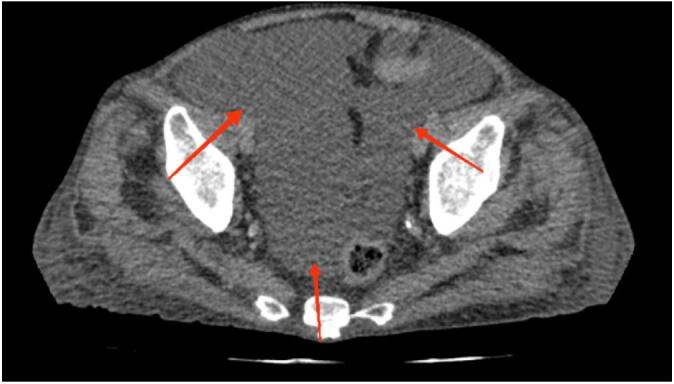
Axial CT scan showing the presence of ascitic fluid in the pelvic cavity, consistent with chylous ascites.
